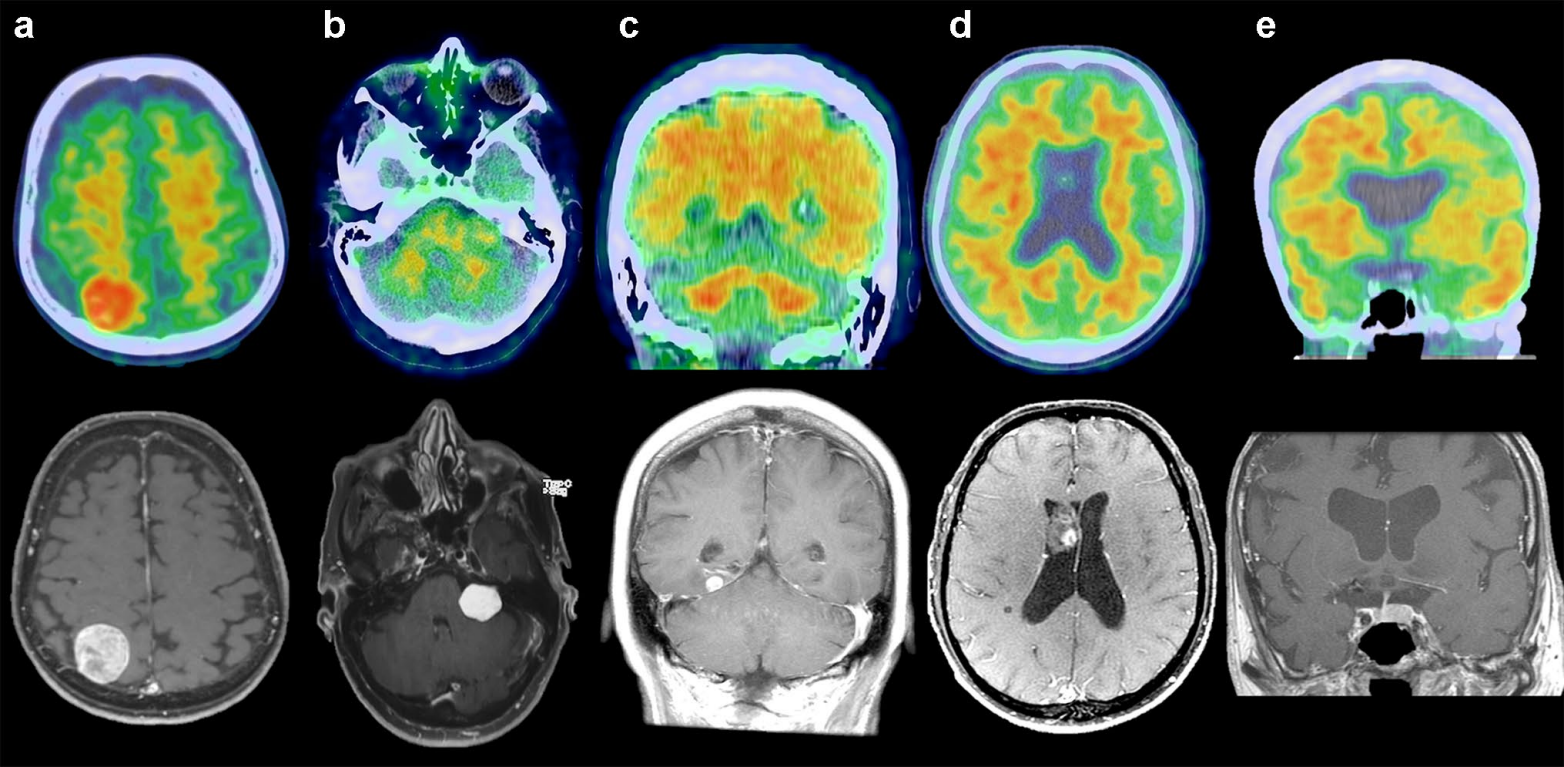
Examples of PiB PET/CT (top row) and T1-weighted post-contrast MRI (bottom row) appearance of meningioma (a), vestibular schwannoma (b), metastatic disease (c), central neurocytoma (d), and pituitary macroadenoma (e). Intense PiB activity is seen only in the meningioma (a)
